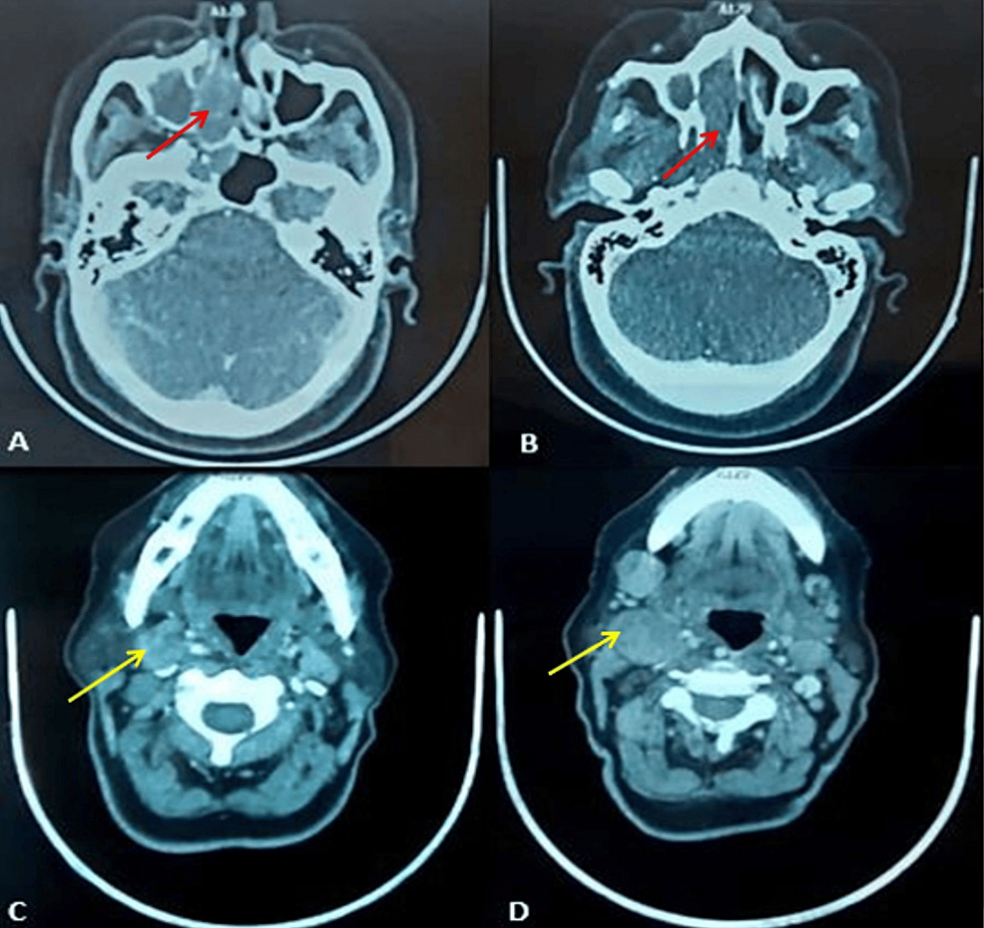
Computed tomography scan images A, B: Axial view showing a mass in the right nasal cavity with lysis of the nasal septum (red arrow). C, D: Axial view showing cervical lymphadenopathy (yellow arrow)
